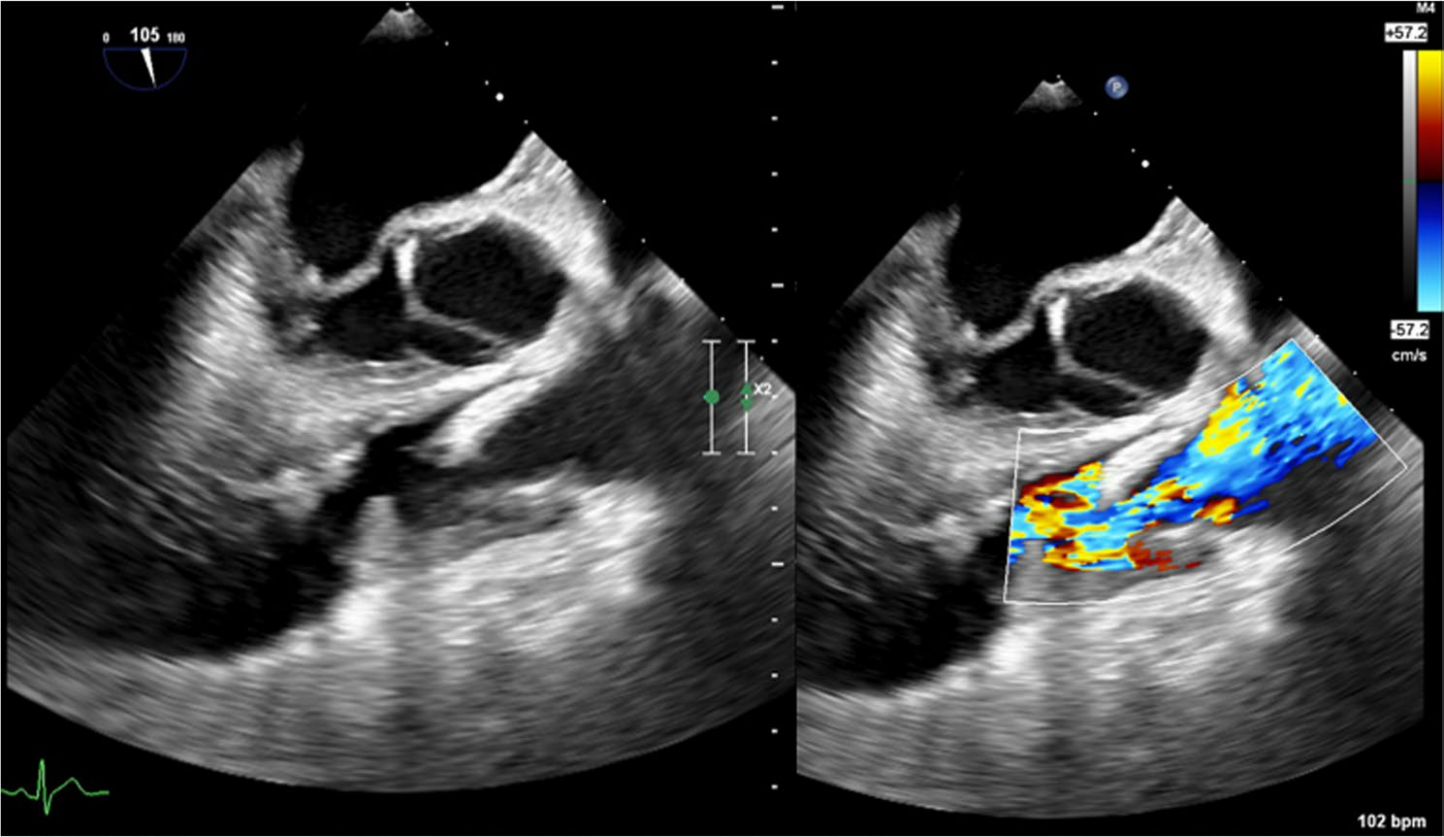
TEE in a mid-esophageal view with simultaneous 2D and color flow doppler demonstrating PV thickening, a 21 mm x 7 mm vegetation, and severe PV regurgitation
